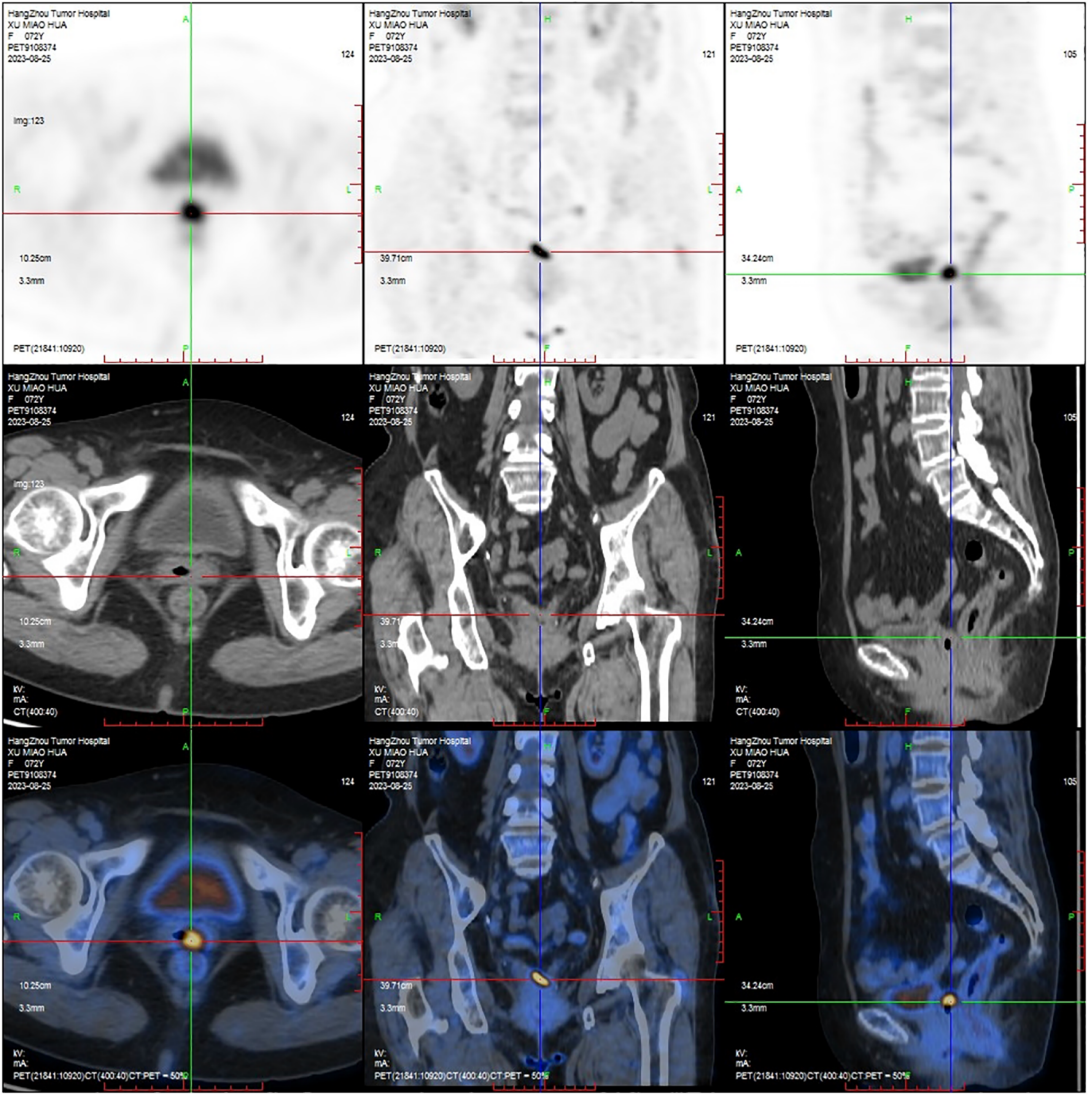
PET-CT depicted the tumor was confined to the apex of vagina.
